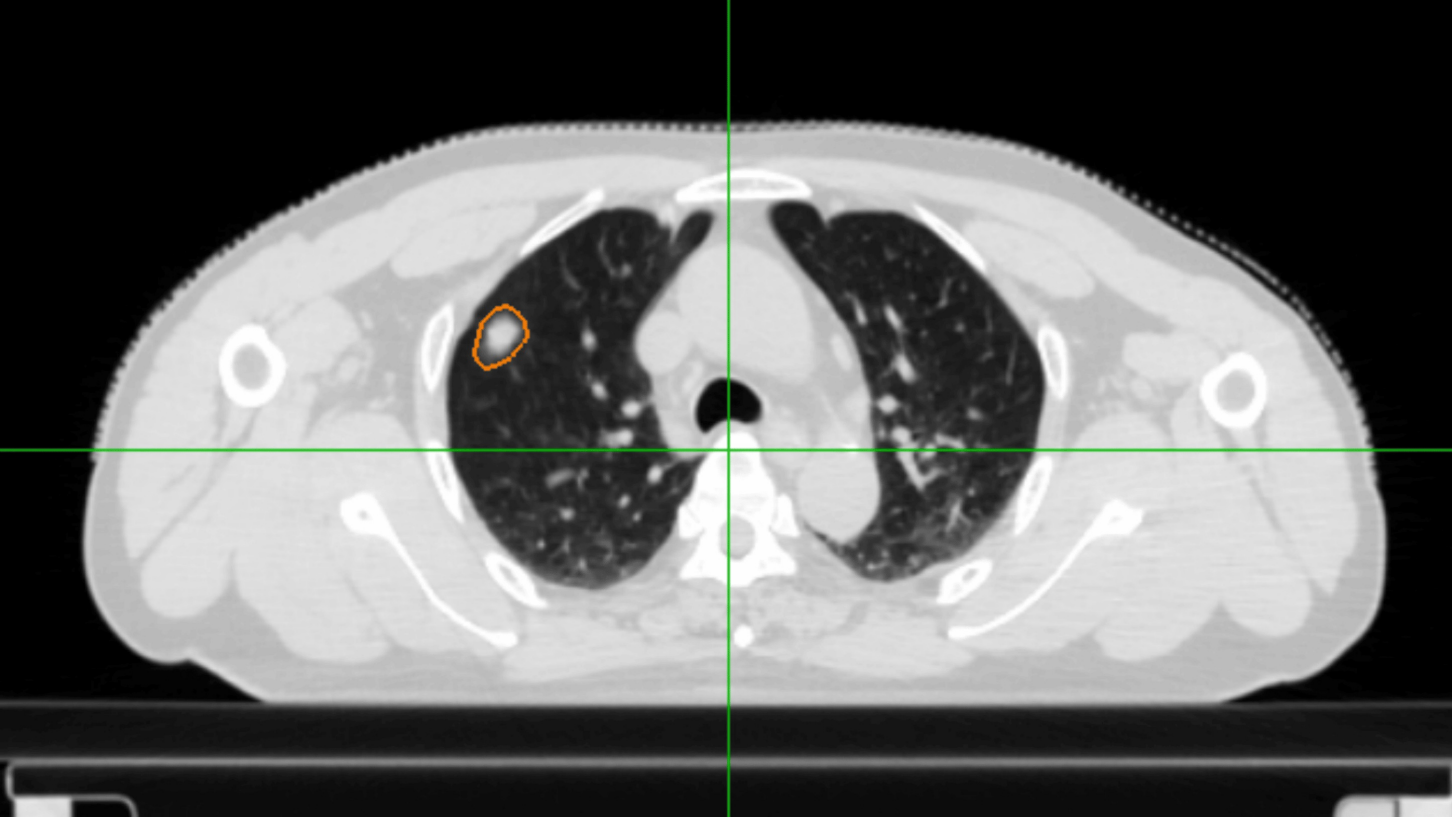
Axial CT showing a 15-mm NSCLC lesion in the left upper lobe CT: computed tomography, NSCLC: non-small cell lung cancer
